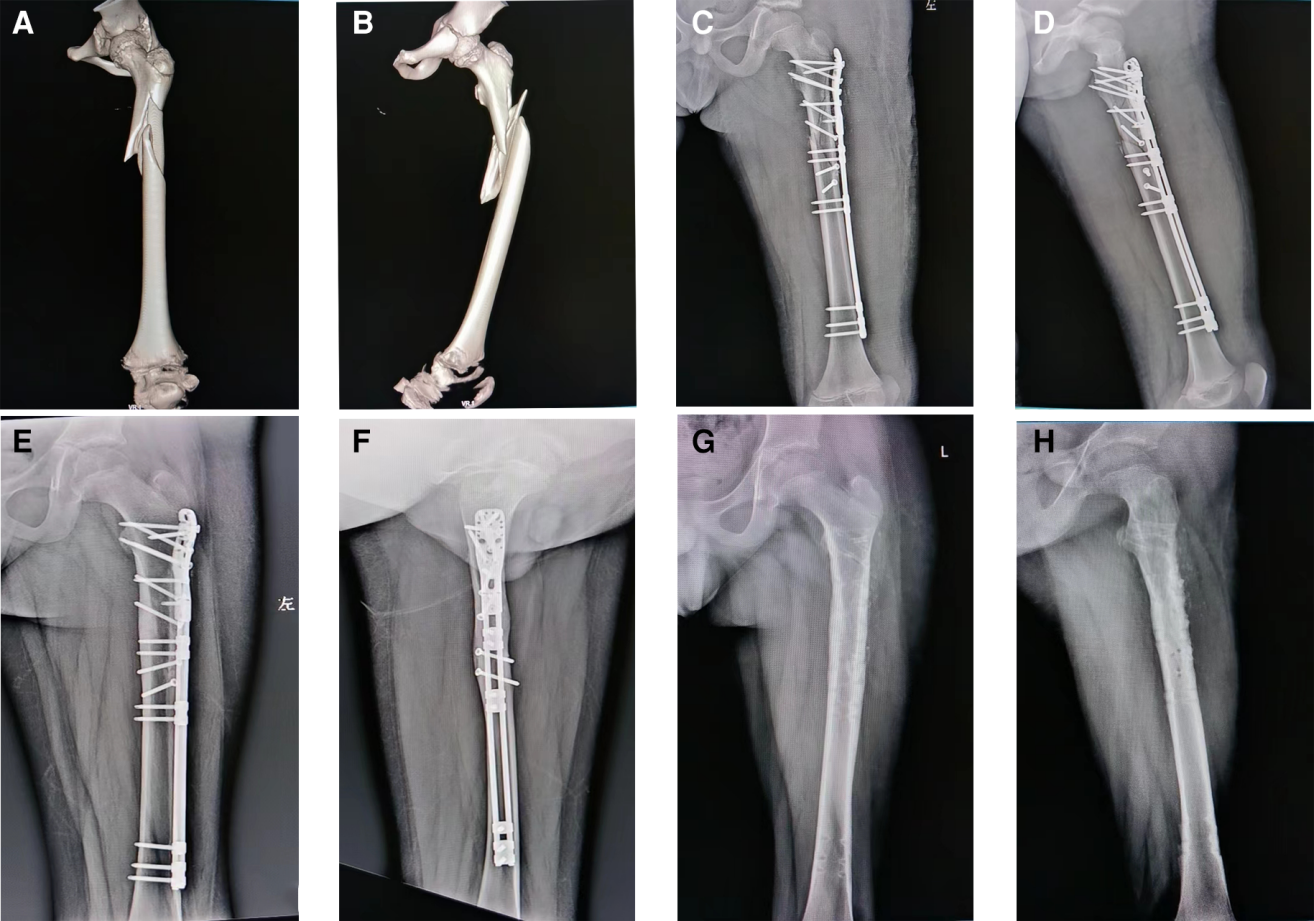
OBS fixation for a complex subtrochanteric femur fracture. Preoperative (A,B) and early postoperative (C,D) radiographs of a 10-year-old boy. The fracture healed at 16 weeks follow-up visit (E,F), and the implant was removed after one year (G,H).
